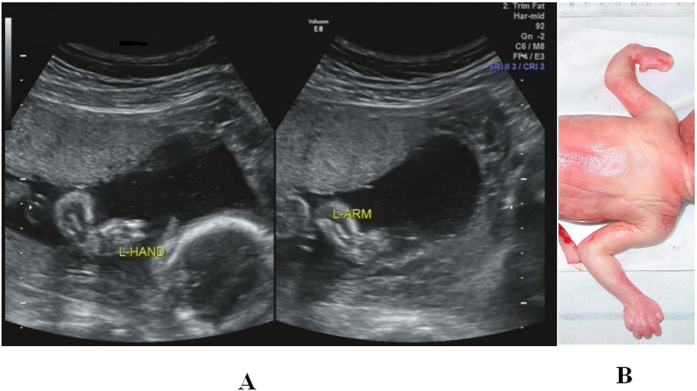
Left hand ectrodactyly at a gestational age of 24 weeks. (A) Two-dimensional sonogram showing the absence of some metacarpal bones in the fetal left hand. (B) Macroscopic view of the fetus, showing shorter fingers and palm abnormalities of the left hand.
